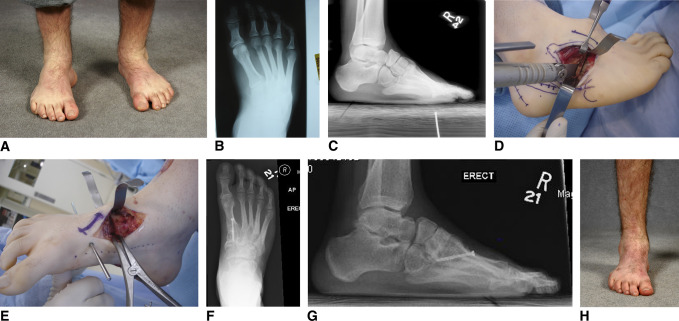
Undercorrection. A–H, Preoperative and postoperative radiographs and photographs of a 17-year-old boy with a history of idiopathic clubfoot and an extensive soft-tissue release at 6 months of age, presenting with recurrent (undercorrected) deformity. The deformity was centered around the midfoot. He was treated with an open plantar fascia release, biplanar midfoot osteotomy with lateral closing and medial opening wedge, dorsiflexion osteotomy of the first metatarsal, anterior tibialis tendon transfer to the third cuneiform, and a peroneus longus to peroneus brevis tendon transfer.
